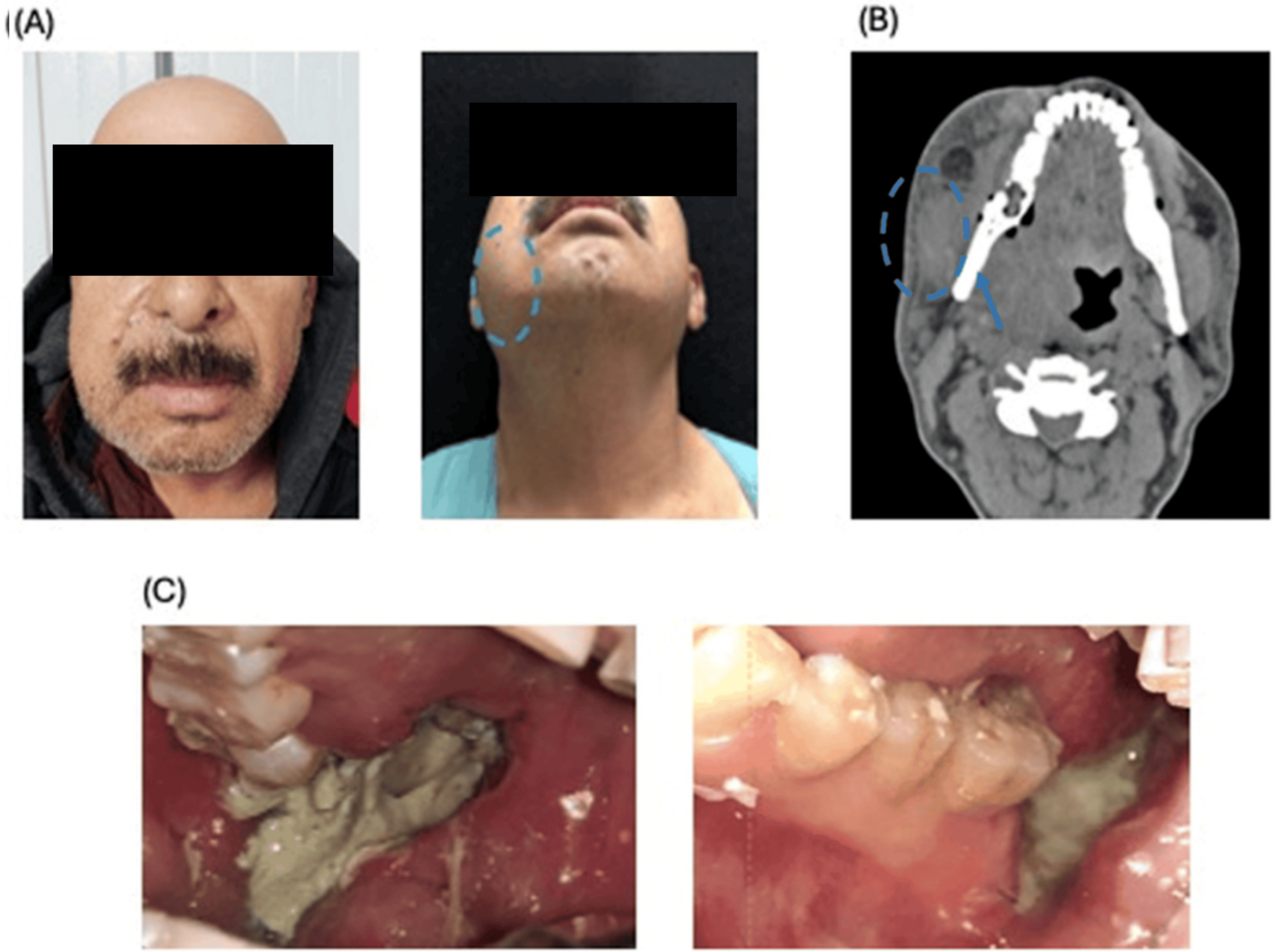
Evaluation of the patient by clinical and imaging modalities A) Images of a patient exhibiting volumetric enlargement in the right oral region. B) CT of the patient. C) Regions of bone exposure and necrotic tissue CT: computed tomography
